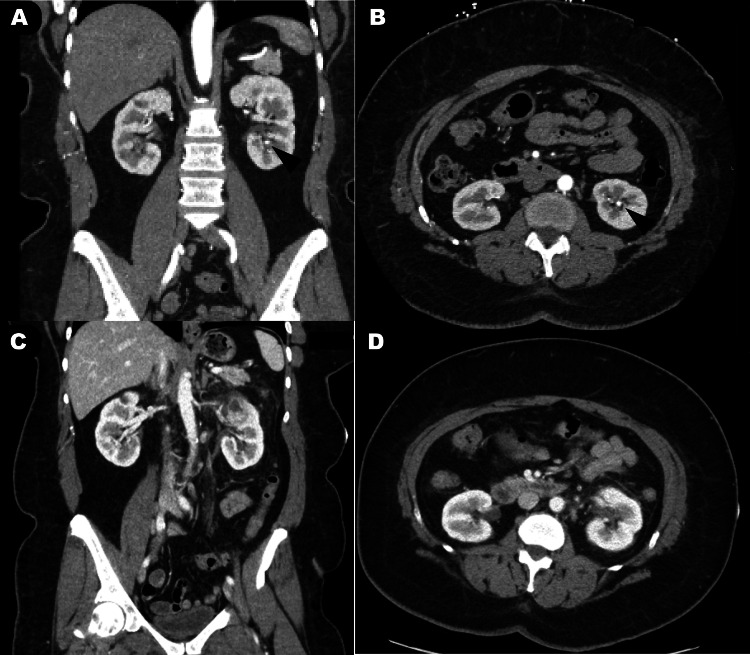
Abdominopelvic CT with contrast ordered by the emergency medicine team for abdominal pain workup. Contrast-enhanced CT imaging of the kidneys. Panels A (coronal) and B (axial) are from an abdominopelvic CT with contrast done a year earlier, and an incidental 5-mm stone was detected with no anatomic anomalies noted. Panels C (coronal) and D (axial) are from the imaging a day before the surgery, ordered by the emergency medicine team.
